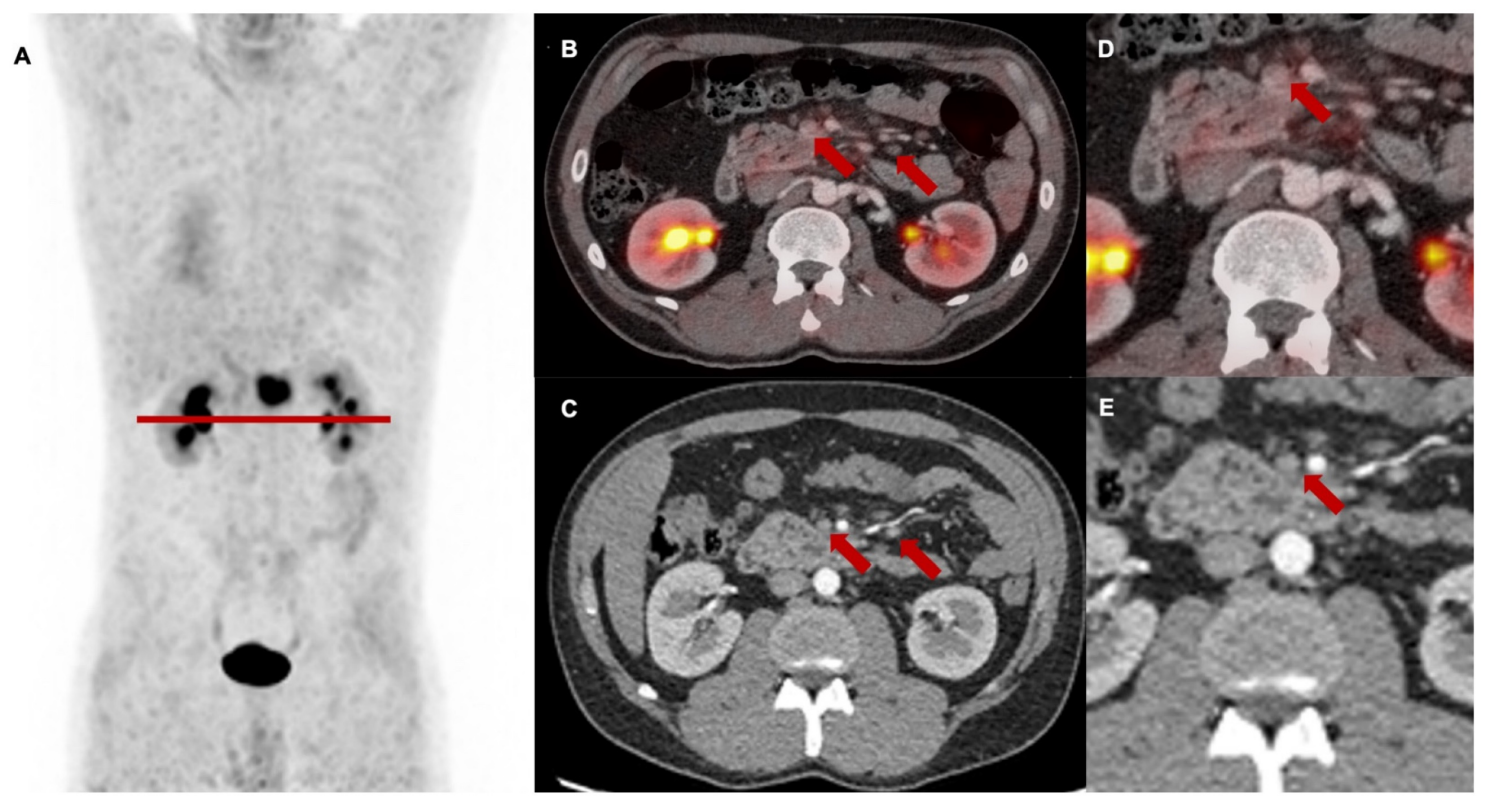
Baseline [^68^Ga]Ga-FAPI-46 PET/CT of a patient with locally recurrent pancreatic adenocarcinoma at the mesentery root. A maximum intensity projection with a red line representing axial slices B-E, B and D transaxial fusion of PET/CT data of the upper abdomen, C and E transaxial*,* corresponding contrast-enhanced CT scan in arterial phase. Suspicious peripancreatic lymph nodes with a short axis diameter over 10 mm (red arrows) without FAPI signal were rated benign and consequently downstaged.
